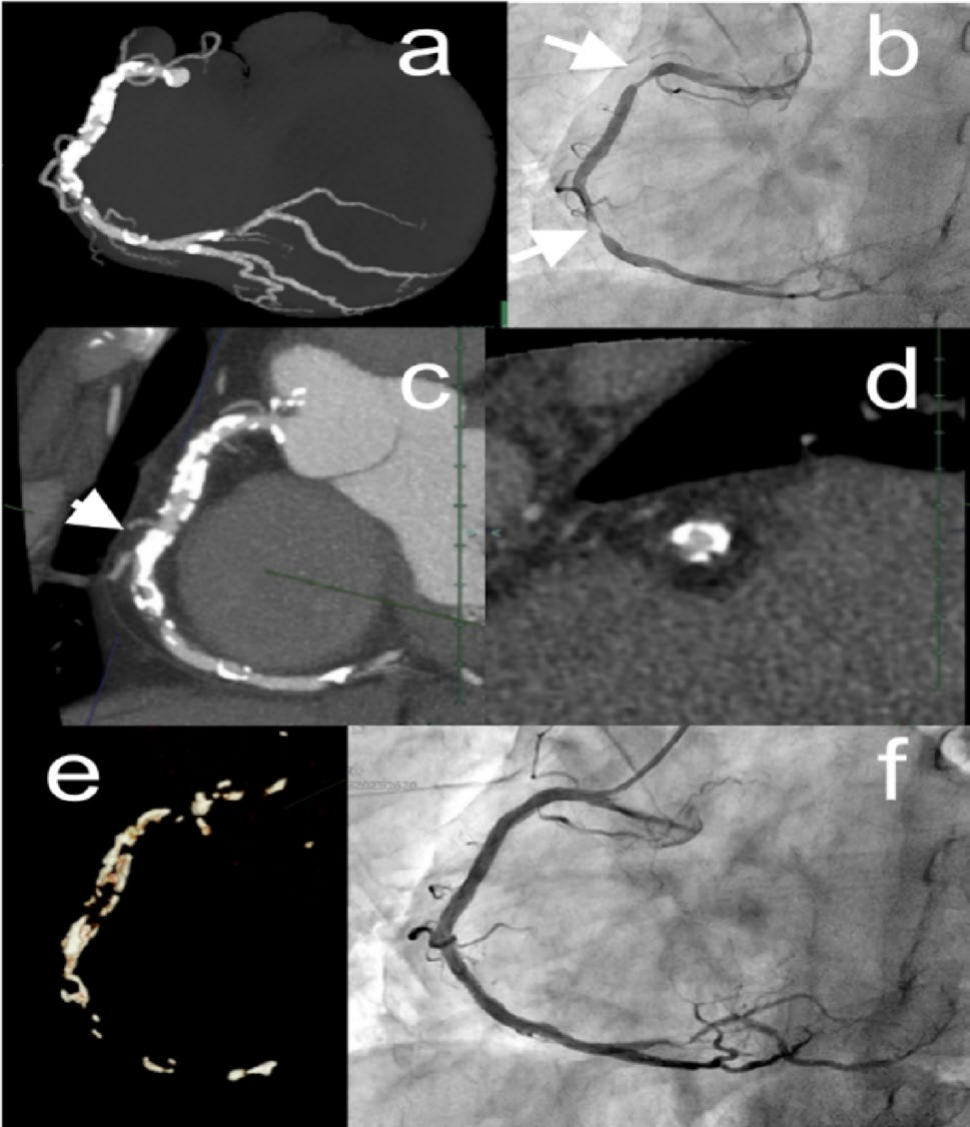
Pre-procedural cardiac CT assessment for slender PCI in a severely calcified Lesion. (a) A full-volume maximal intensity projection shows a calcified right coronary artery; however, precise evaluation of the luminal stenosis is difficult. (b) Coronary angiography shows proximal and mid lesions (arrows). (c) Thin-slab maximum intensity projection shows the calcified coronary artery. (d) The corresponding short-axial view at the most severely calcified lesion (arrowhead) reveals that the calcified plaque is non-circumferential and is divided into three segments. (e) 3D visualization of the calcified plaque shows that calcification is not circumferential. (f) Coronary angiography after the intervention shows the successful placement of two drug-eluting stents using a slender 5-Fr guiding catheter. *3D* three-dimensional, *CT* computed tomography, *Fr* French, *PCI* percutaneous coronary intervention
